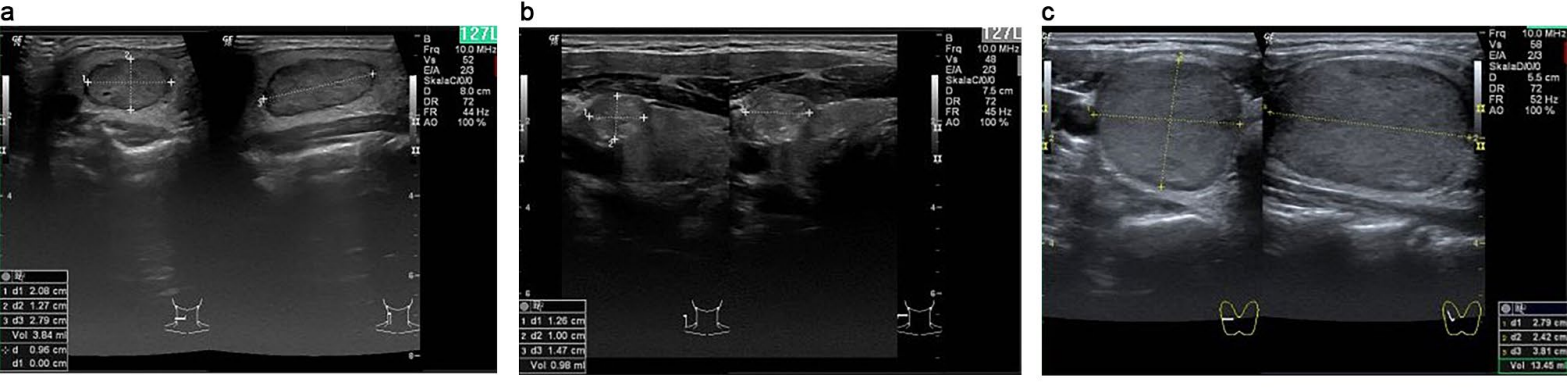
Sonographic appearances of follicular and parafollicular thyroid nodules. a) MTC right lobe, b) PTC right lobe, c) FTC right lobe. MTC: medullary thyroid carcinoma, PTC: papillary thyroid carcinoma, FTC: follicular thyroid carcinoma.
